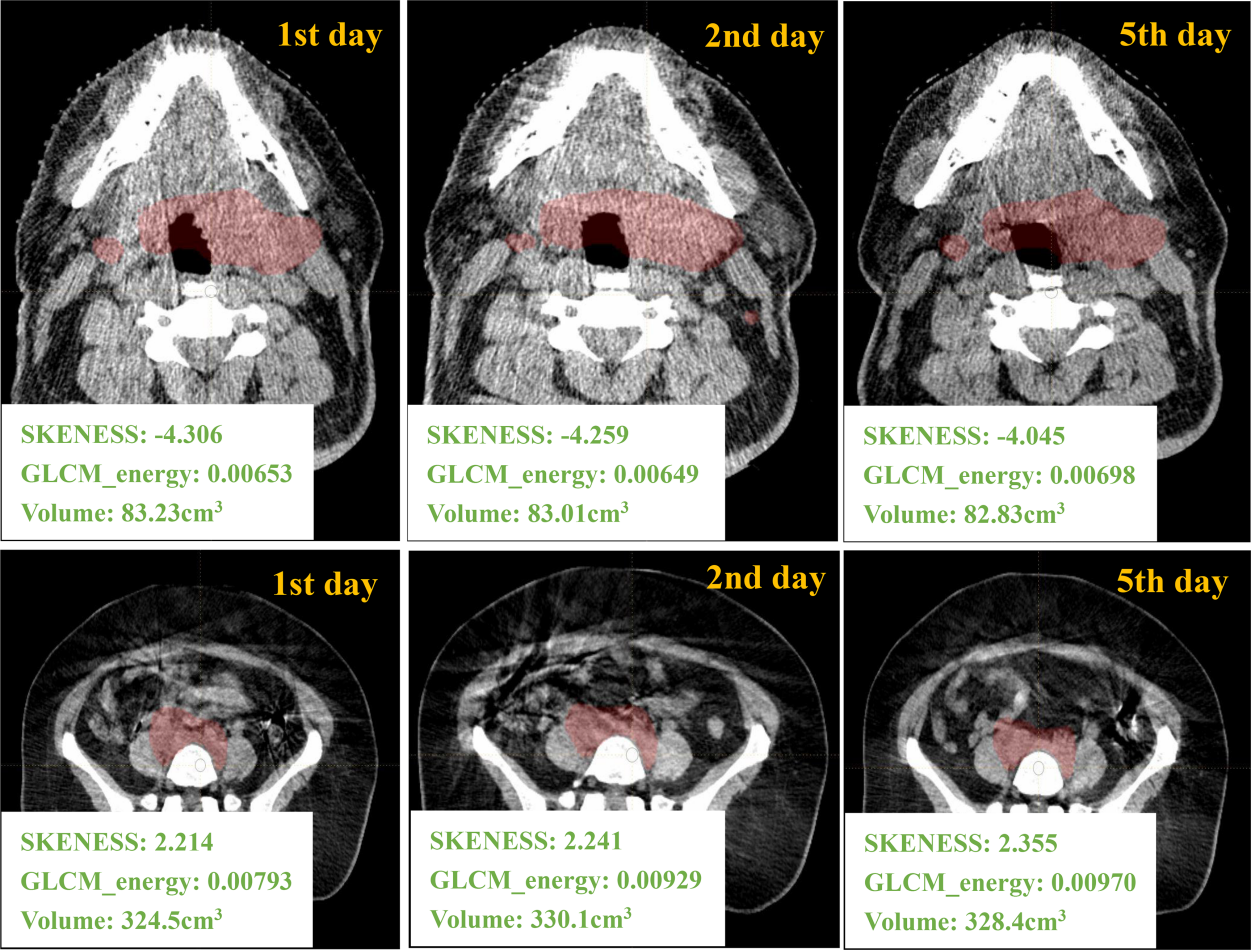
Different days of CBCT images and the corresponding radiomics/volume values from one H&N ROI and one pelvic ROI.
